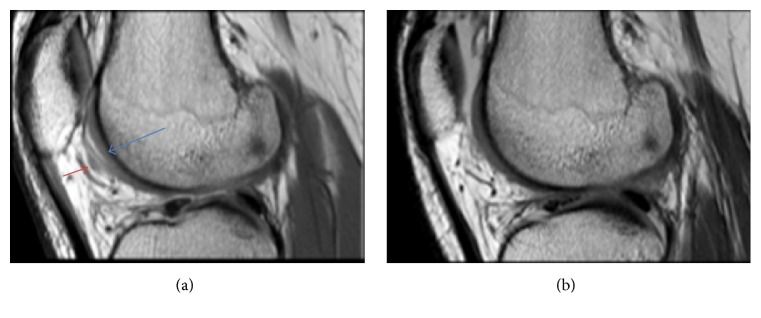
(a) Sagittal FIESTA image showing a grade 2 cartilage defect (blue arrow) with FNMHF (red arrow). (b) Follow-up MRI 2 years later showing healed cartilage defect and absent synovial thickening.
